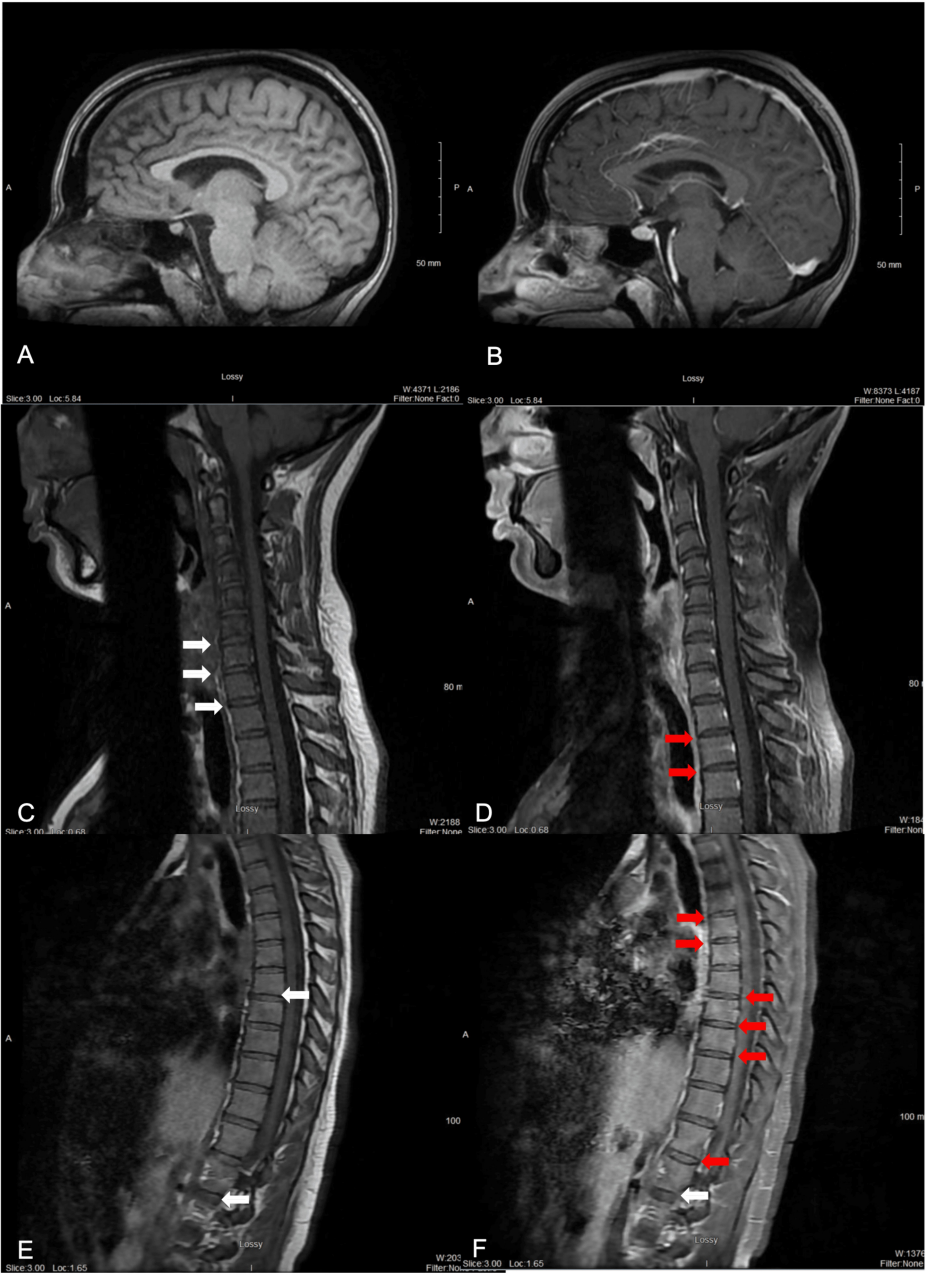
Repeat neuraxial MRI with and without contrast, sagittal view. Image taken three months after symptom onset. (A) Brain MRI without contrast; (B) brain MRI with contrast; (C) cervical MRI without contrast; (D) cervical MRI with contrast; (E) thoracic MRI without contrast; (F) thoracic MRI with contrast. White arrows: disc dehydration. Red arrows: possible bamboo spine.
